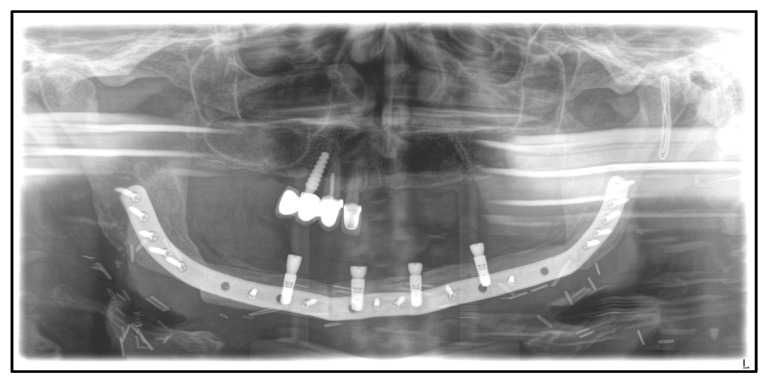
Dental X-ray after implantation of four endosseous implants in the neo-mandible for dental rehabilitation and extraction of the upper first incisor on the right, August 2021.
